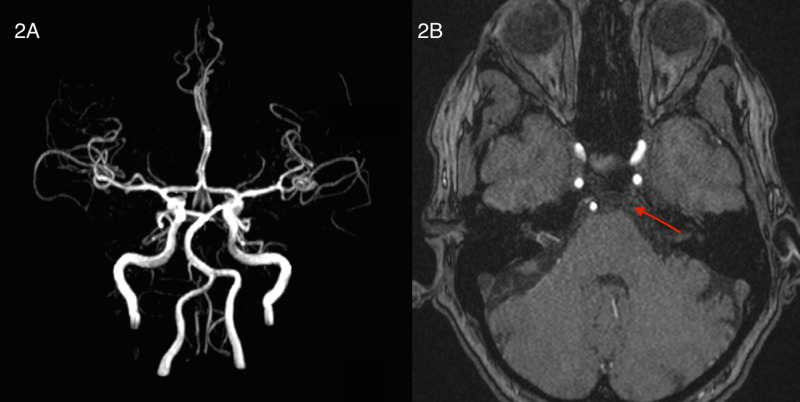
MRI. 2A: Magnetic Resonance Angiography (MRA) - Maximum Intensity Projection (MIP) images fail to reveal a left vertebral dissection. 2B: T1 weighted axial images reveal a signal void of the left V4 vertebral artery (red arrow) MRI: Magnetic Resonance Imaging
